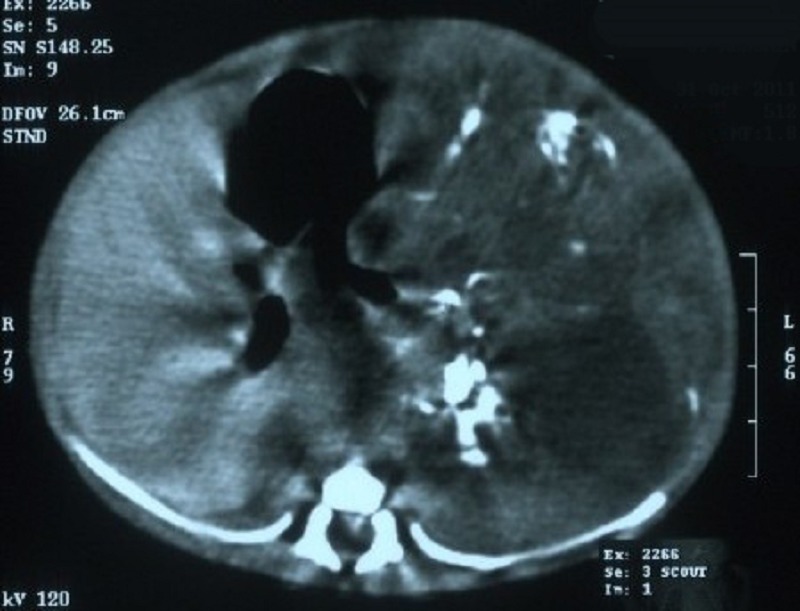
Figure 1: CT scan of the abdomen demonstrated a large lobulated heterogeneous mass with solid and cystic components with calcification.
